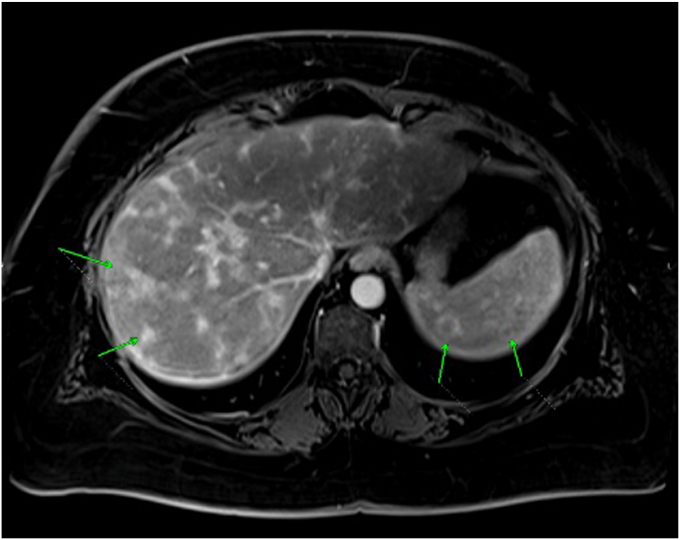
T1 axial view contrast enhanced MR image of the abdomen showing enhancing hepatic lesions and peripherally enhancing splenic lesions. *MR* = magnetic resonance.
